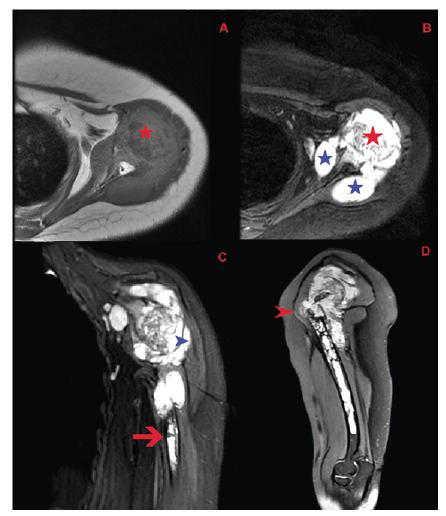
In the patient’s initial shoulder MRI , axial images show an intramedullary lesion within the humeral head causing bone destruction (red asterisks). A. On T1-weighted images, the lesion demonstrates intermediate signal intensity similar to muscle. B. On PD images, it appears hyperintense. An increased amount of reactive fluid is observed in the glenohumeral joint space (blue asterisks). C. On coronal PD images, the lesion extends distally along the humeral diaphysis (red arrow). In addition, lesions with a multicystic nature and internal septations are observed within adjacent muscle compartments, suggesting muscular involvement (blue arrowhead). D. On sagittal PD images, a pathological fracture is seen at the humeral head with disruption of the axis at this level (red arrowhead).
